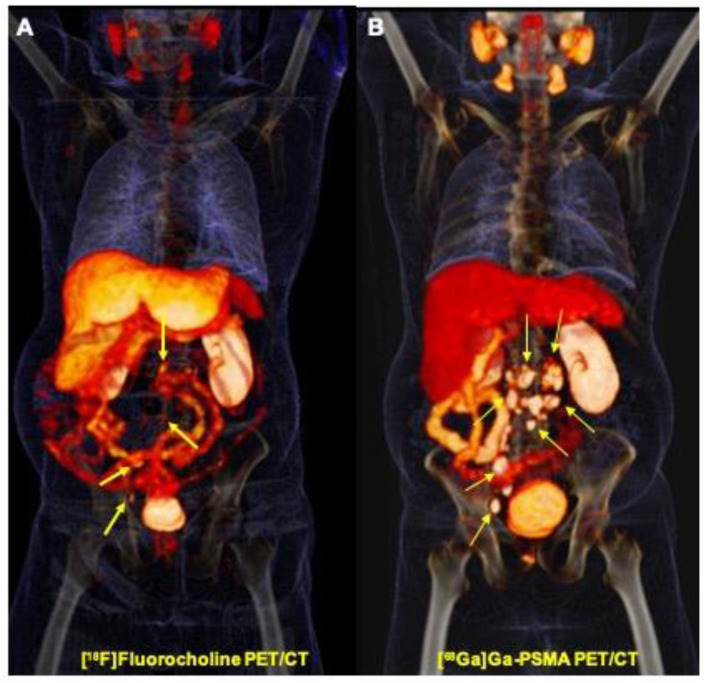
Prostate cancer recurrence: superior value of [^68^Ga]Ga-PSMA PET/CT comparing to [^18^F]Fluorocholine PET/CT in the detection of lymph node metastases (Gleason score 8, PSA: 25 ng/mL). (A) [^18^F]Fluorocholine PET/CT (volume-rendered maximum intensity projection (MIP)) shows multiple small faint [^18^F]Fluorocholine uptakes in the metastatic abdominal lymph nodes (arrows). (B) [^68^Ga]Ga-PSMA PET/CT (volume-rendered MIP) shows multiple intensive [^68^Ga]Ga-PSMA positive abdominal lymph nodes, clearly superior to [^18^F]Fluorocholine PET/CT (arrows).
